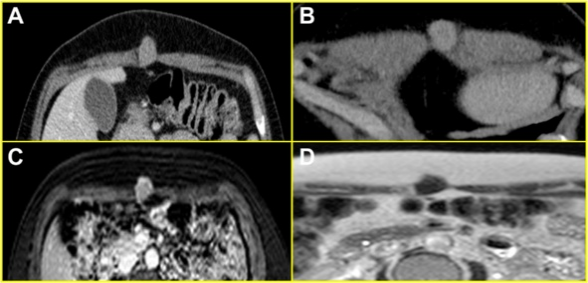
Computed Tomography and Magnetic Resonance Imaging. a Axial contrast-enhanced CT image, obtained during the venous phase, showing the nodular lesion, located within the right rectus abdominis muscle. The lesion is homogeneously enhancing, demonstrating a vascularity higher than that of the adjacent muscular tissue; b On axial MR T2-weighted the lesion is hypointense compared with adjacent muscle; this finding is indicating a tissue with high cellularity; c pre-contrast T1-weighted image and contrast-enhanced fat-suppressed T1 weighted images d confirm the vascularity of the lesion
